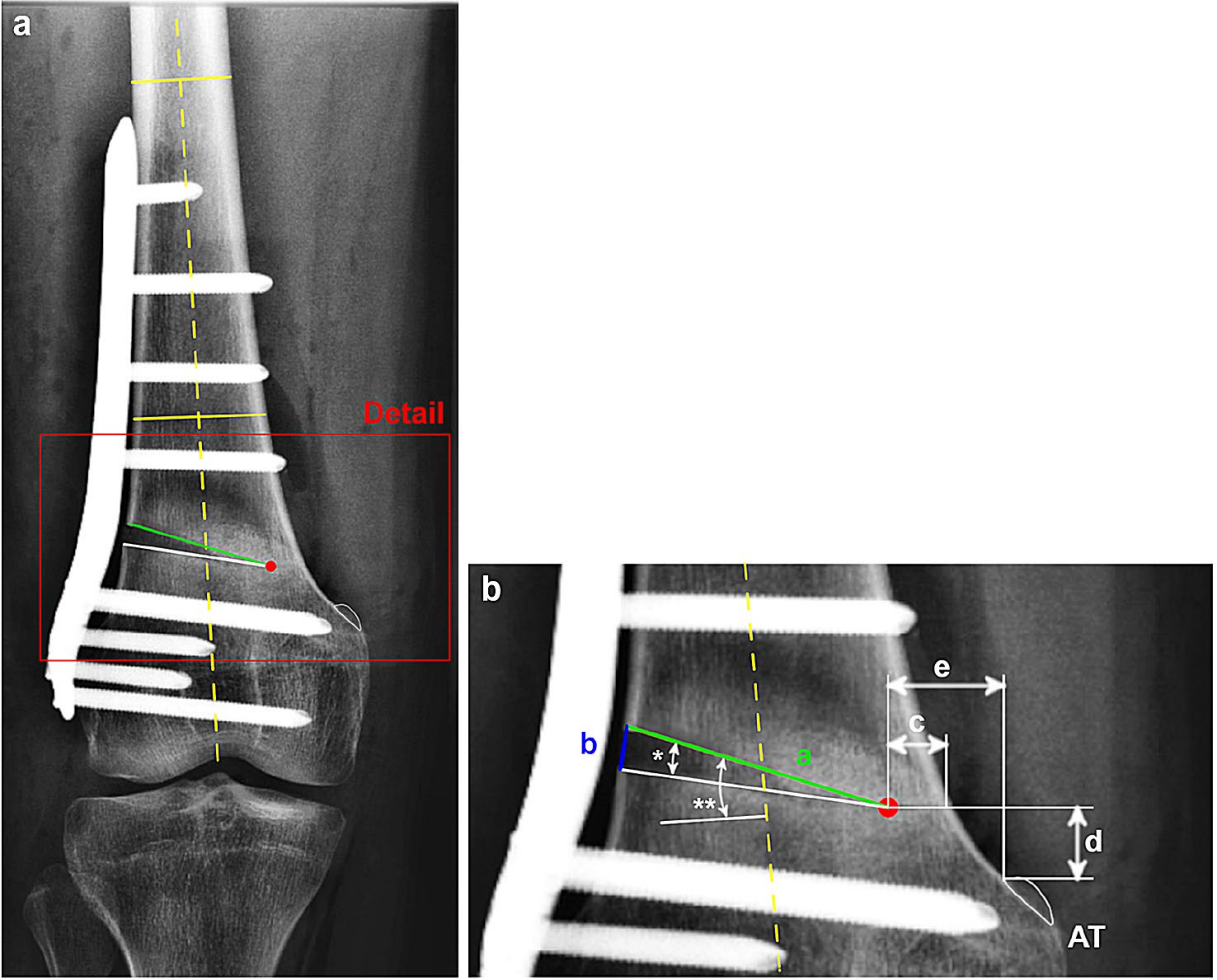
Postoperative radiographic measurements. a Standard anterior–posterior radiograph of a right knee after lateral open wedge distal femoral osteotomy using a TomoFix™ (DePuy Synthes, Raynham, MA, USA) locking compression plate. b Detailed view of the osteotomy gap with the corresponding measurements. Red dot, osteotomy hinge; *AT* adductor tubercle (encircled in white); Yellow solid lines, medial–lateral connection of the femoral cortical bone; Yellow dashed line, anatomical axis of the femoral diaphysis, defined as the line connecting the midpoints of the two yellow solid lines; Green line (distance “a”), length of the osteotomy gap; Blue line (distance “b”), height of the osteotomy gap; Distance “c”, horizontal distance between the medial cortical bone and the osteotomy hinge; Distance “d”, vertical distance between the proximal margin of the AT and the osteotomy hinge; Distance “e”, horizontal distance between the proximal lateral margin of the AT and the osteotomy hinge; *(angle alpha), wedge angle between the proximal and distal osteotomy plane; (angle beta), slope of the osteotomy defined as the angle between a perpendicular line to the anatomical axis of the femur and the proximal osteotomy plane
